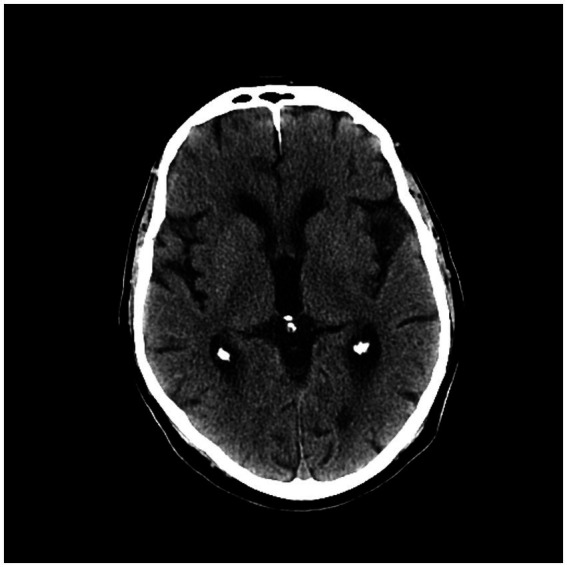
Second CT scan showing left posterior cortex (PIC) ischemic lesion.
